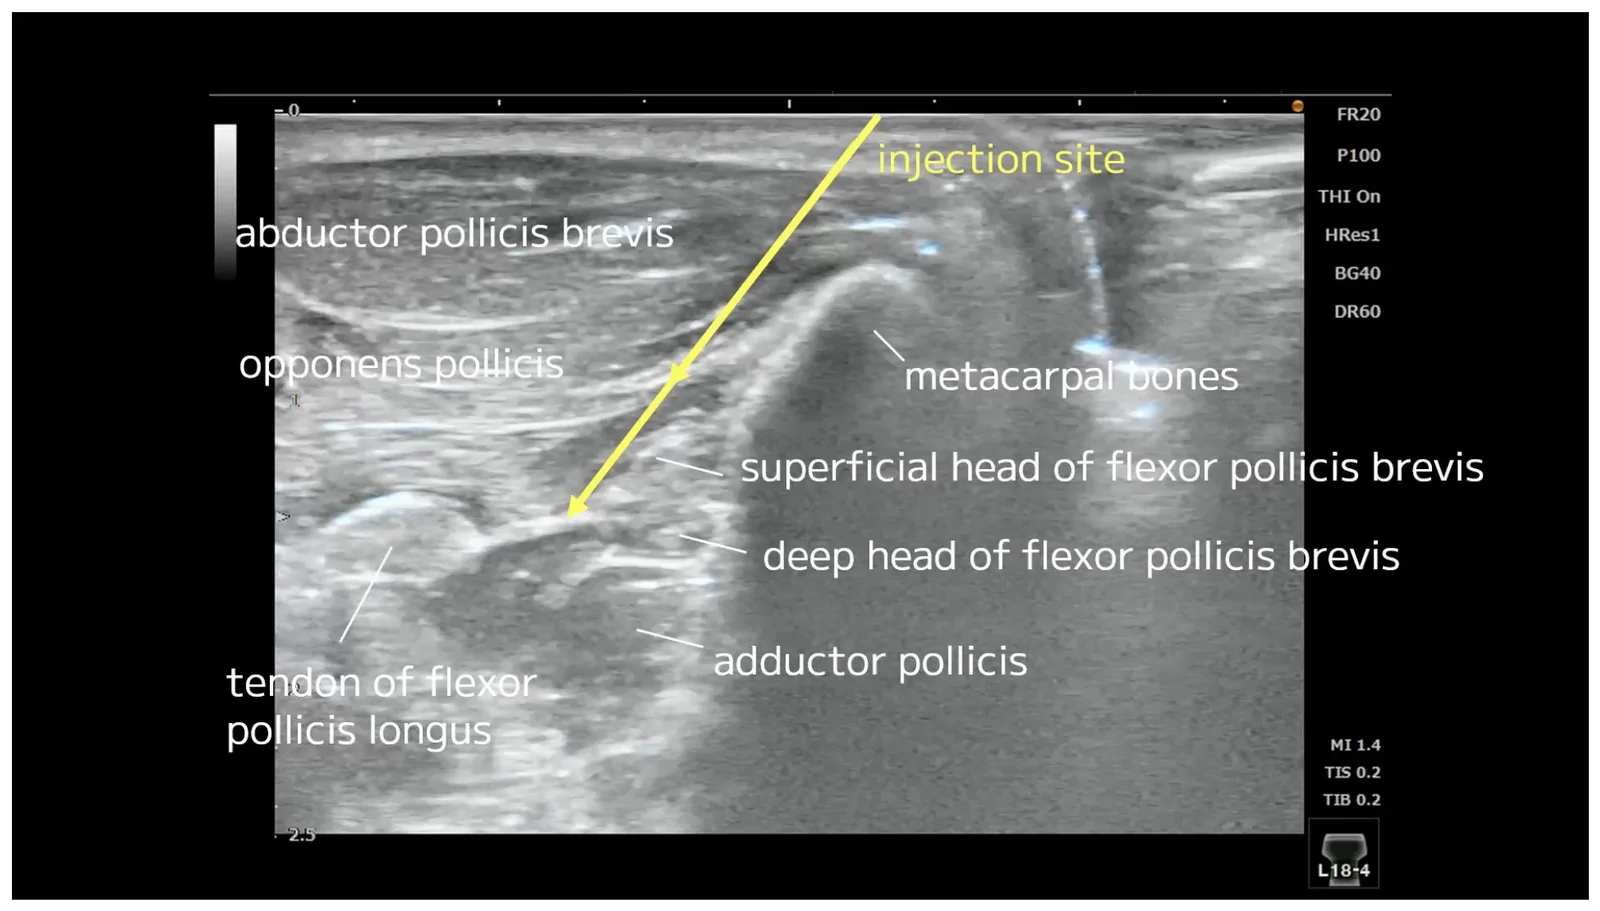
Point 10—thenar muscles: ultrasound-guided fascia hydrorelease; yellow arrow indicates the injection site.
